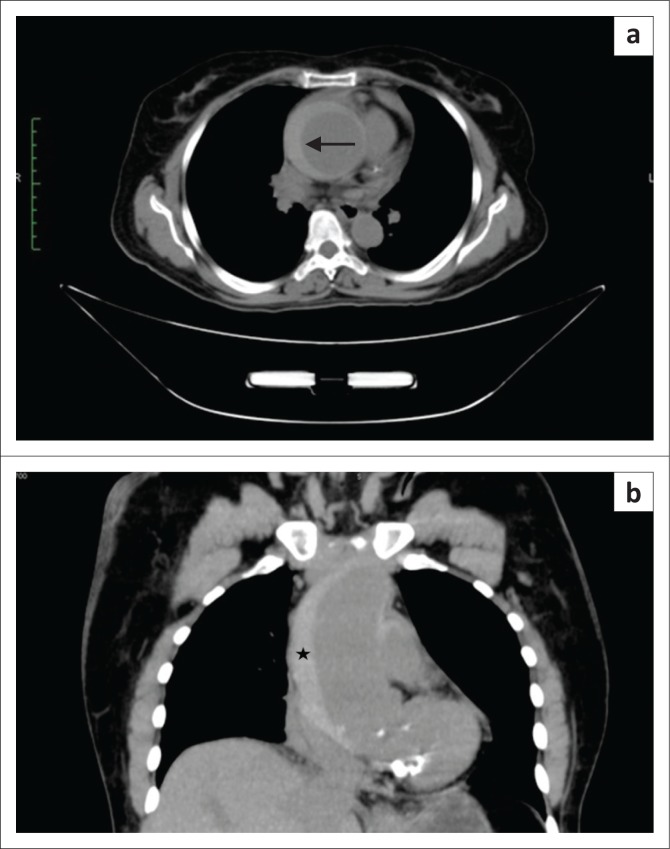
Stanford type A intramural haematoma (IMH): (a) Unenhanced axial computed tomography (CT) scan showing hyperdense crescentic aortic wall thickening involving the ascending aorta (arrow); (b) Stanford type A IMH. Unenhanced coronal CT scan showing crescentic hyperdense aortic wall thickening (*) compressing the lumen.
